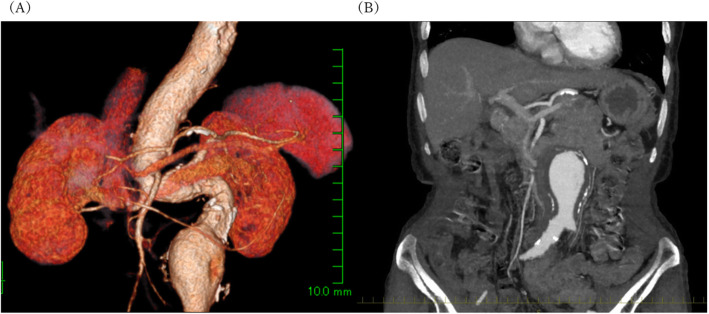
Preoperative computed tomography. A An aneurism, 47 mm in diameter, under the renal artery. B The aneurism contained a thick intramural thrombus and the lumbar arteries were almost occluded
